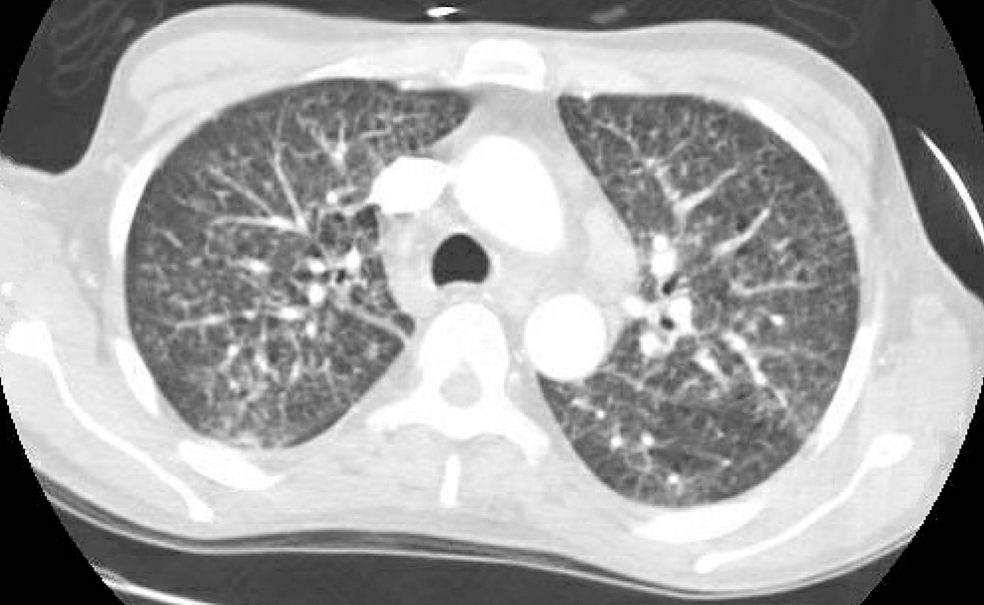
Reticulonodular opacities shown in CT of the chest
